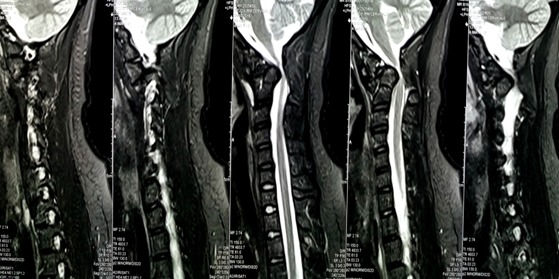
Cervical spinal MRI showing C1-C2 dislocation with compression of the bulbo-medullary junction
